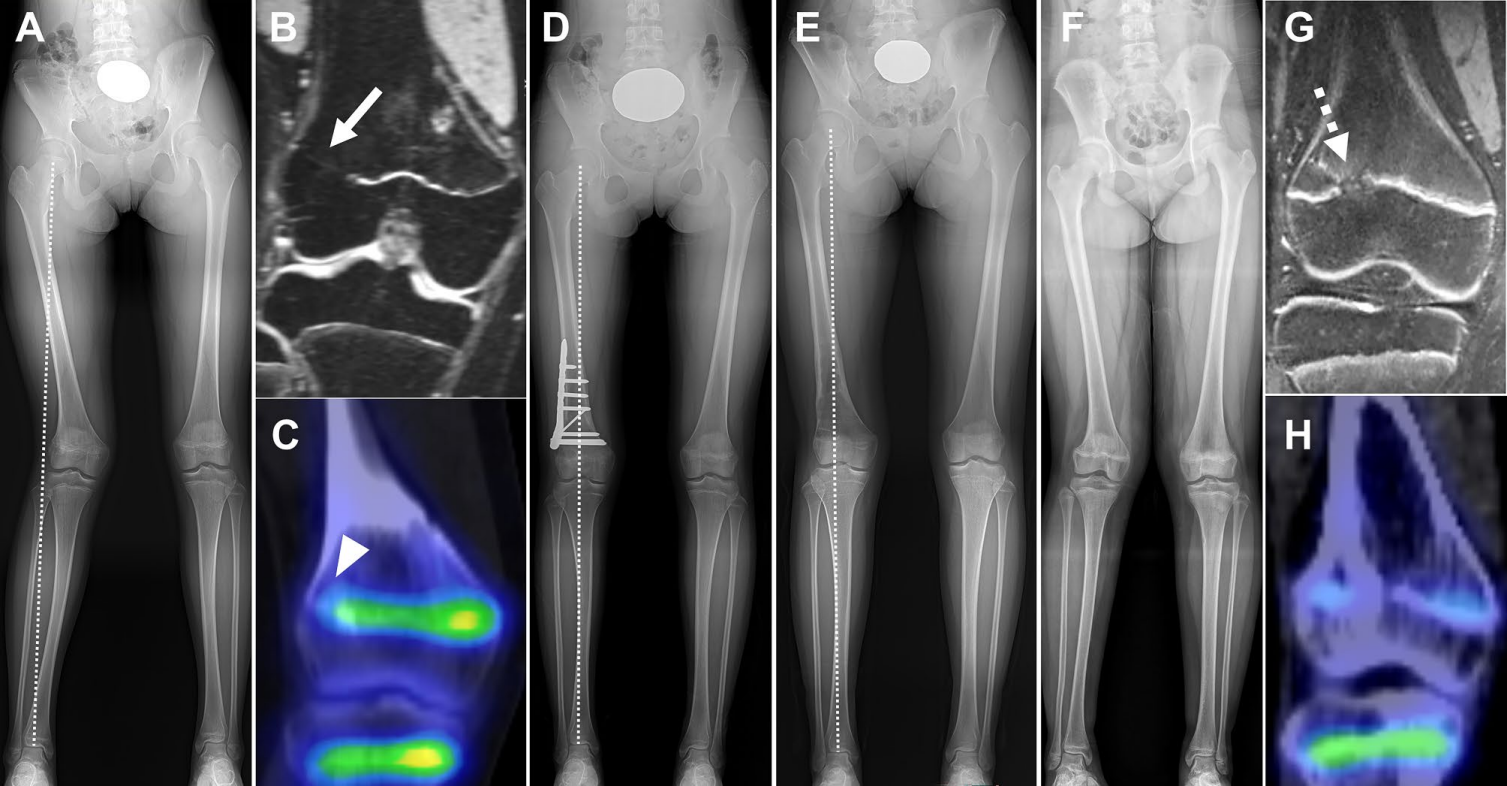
Bone SPECT/CT-based treatment planning for growth disturbance of the long bone. An 11-year-old girl presented with posttraumatic genu valgum and shortening of the right leg (A). MRI reveals a peripheral bone bridge (arrow) at the lateral distal femoral physis (B). There is no uptake of ^99m^Tc-DPD at the bone bridge site (arrowhead) on bone SPECT/CT (C); however, physeal activity remains in the surrounding physis (C). She underwent arthroscopy-assisted bone bridge resection and opening-wedge distal femoral varus osteotomy (D). No aggravating leg length discrepancy was noted until post-operative 2.3 years, indicating that the right leg had undergone longitudinal growth (E). Another 11-year-old girl presented with posttraumatic genu valgum and shortening of the right leg (F). MRI reveals a slightly laterally located bone bridge (dashed arrow) at the distal femoral physis (G). On bone SPECT/CT, almost no remaining physeal activity is apparent in the entire right distal femoral physis (H). Based on these findings, she underwent distraction osteogenesis rather than epiphysiodesis or bone bridge resection
